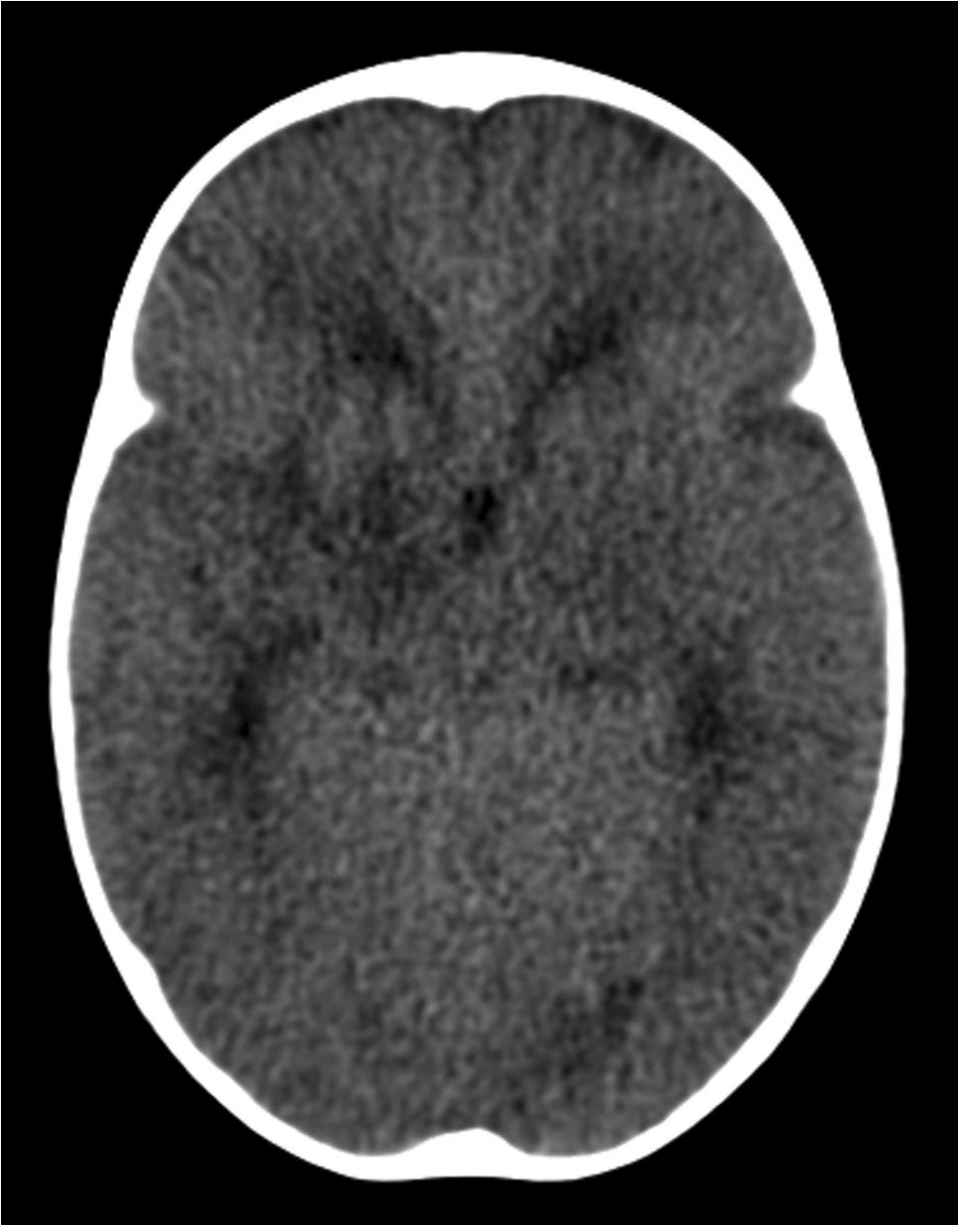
Axial non-contrast computed tomography of the brain in a 7-year-old boy with tuberculosis shows infarcts in the “TB zone” of the bilateral medial lenticulostriate and thalamo-perforating vessels (head of the caudate nucleus, anterior limb of the internal capsule and the anteromedial thalamus)
